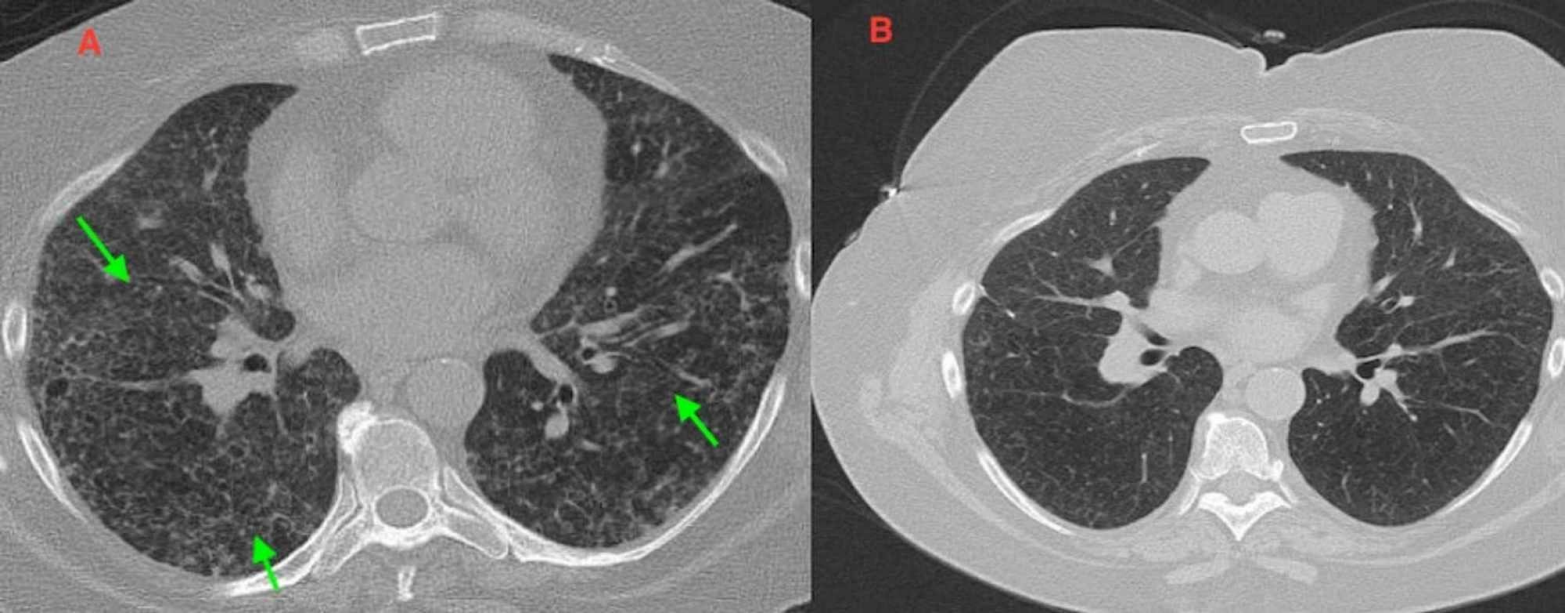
(A) CT chest shows diffuse reticular and nodular interstitial opacities, and ground-glass infiltrates, upper and mid lung predominant with lower lobes involvement (arrows). (B) Follow-up CT scan at three months shows interval improvement in reticulonodular and ground-glass infiltrates compared to (A).
